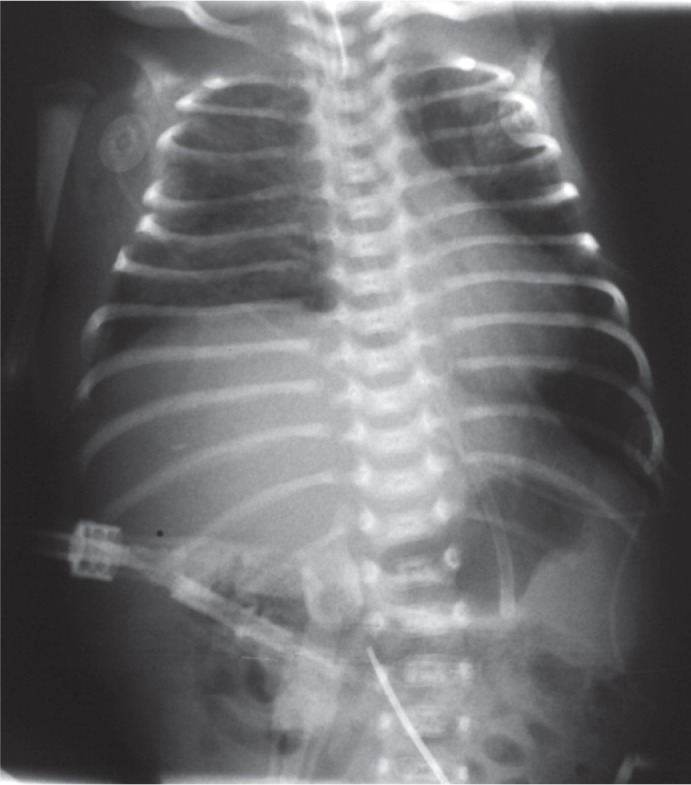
Chest x-ray demonstrates high position of the right diaphragm due to postoperative paralysis.
